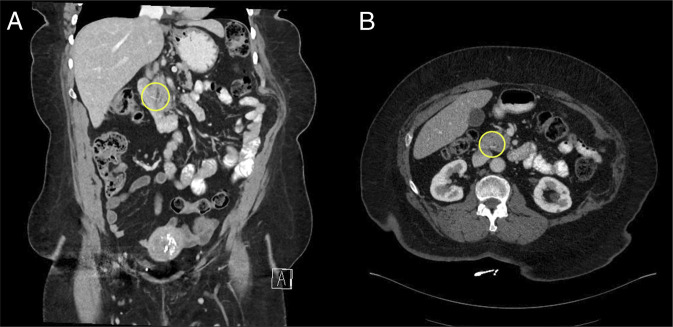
Computed tomography image showing linear hypodensity traversing the wall of the second portion of the duodenum and extending to the pancreatic head and traversing the pancreatic duct (A) coronal plane (B) axial plane.
